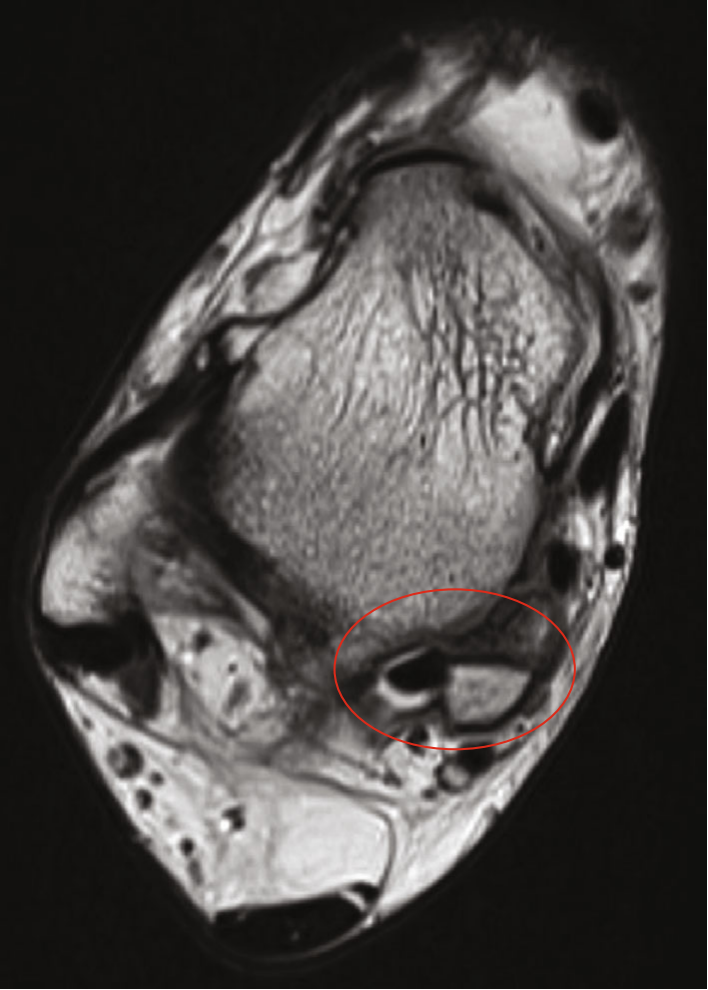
Axial slice of T2 MRI of right ankle demonstrating fluid enhancement around the FHL tendon with the adjacent fractured posteromedial talus fragment.
